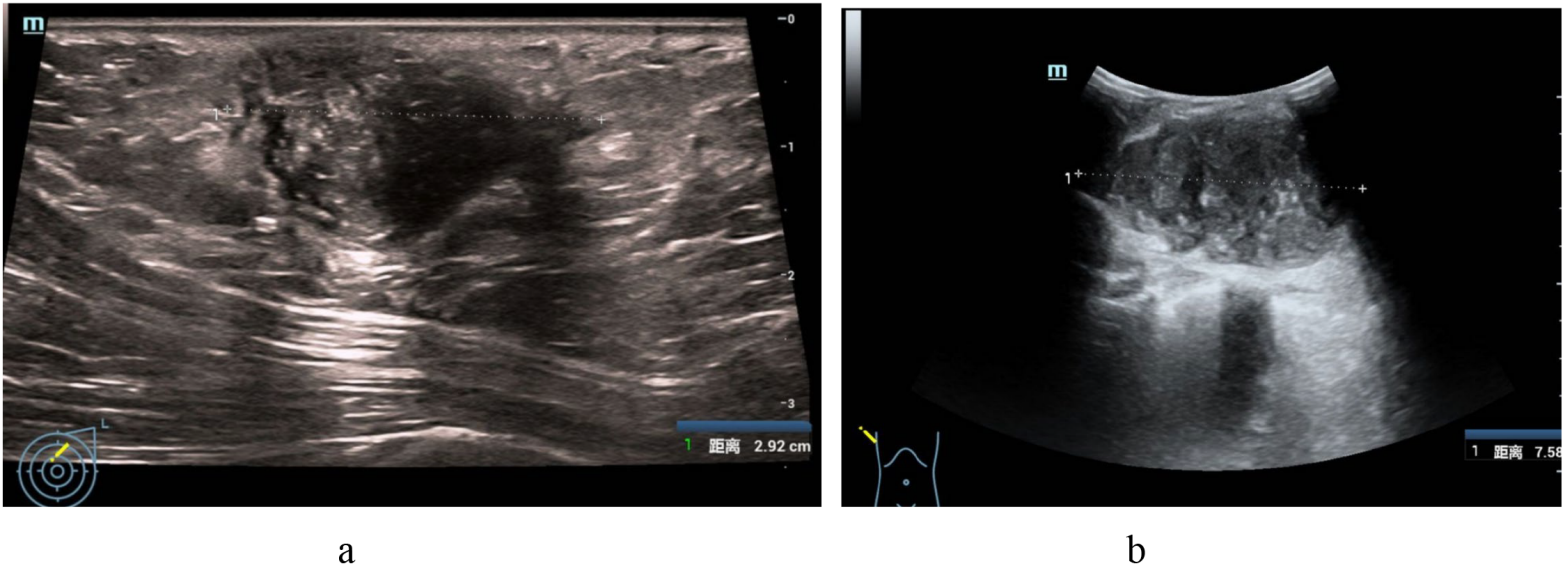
Ultrasound findings. (a) Light breast: A hypoechoic mass with relatively well-defined borders, irregular morphology, and heterogeneous internal echogenicity was identified within the fatty layer at the 1 o’clock position, demonstrating intralesional vascularity. (b) Right breast: Multiple hypoechoic masses with relatively well-defined borders and moderately irregular morphology were observed in the upper quadrant, exhibiting internal vascularity.
